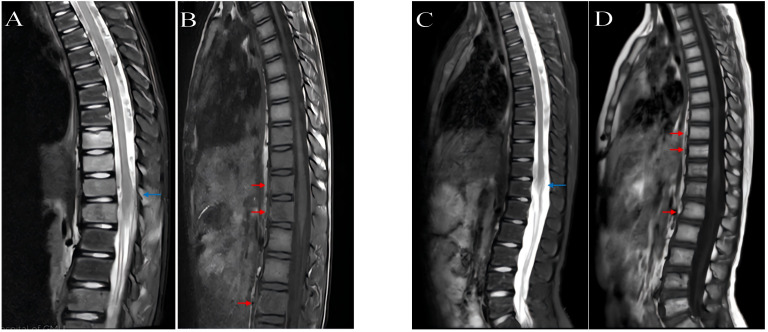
Spinal MRI findings in a 9-year-old boy with *TTMV::RARA* APL. (A) Sagittal T2-weighted image shows severe spinal cord compression at the T10–11 level (blue arrow) with mild intramedullary T2 hyperintensity, consistent with compressive edema, and severe spinal canal narrowing. (B) Sagittal T1-weighted image demonstrates diffuse low T1 marrow signal in the T10 (upper arrow), T12 (middle arrow), and L3 (lower arrow) vertebral bodies with loss of normal fatty marrow signal, consistent with marrow involvement. (C) Sagittal T2-weighted image at the 10-month post-treatment follow-up shows marked improvement of spinal canal narrowing at T10–11 and normalization of spinal cord signal (blue arrowhead), with resolution of compressive edema. (D) Sagittal T1-weighted image at follow-up shows interval fatty marrow reconstitution in the T10, T12, and L3 vertebral bodies (red arrowheads), with patchy hyperintensity replacing the pretreatment diffuse hypointense marrow pattern.
